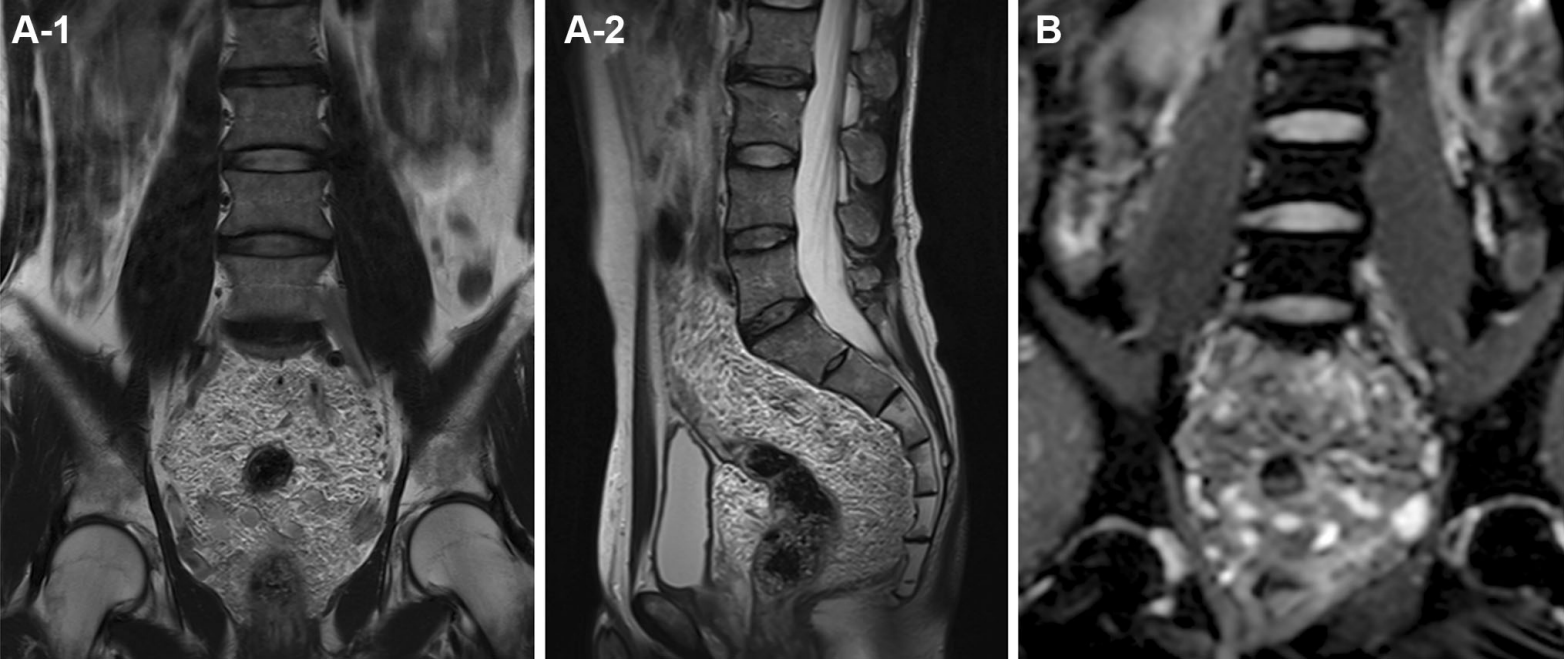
Magnetic resonance imaging. T2-weighted imaging (a1 coronal plane, a2 sagittal plane) shows thickened wall of the sigmoid colon and rectum and fatty tissue hyperplasia. Diffusion-weighted imaging (b coronal plane) show tortuous and dilated vessels in the fatty tissue
